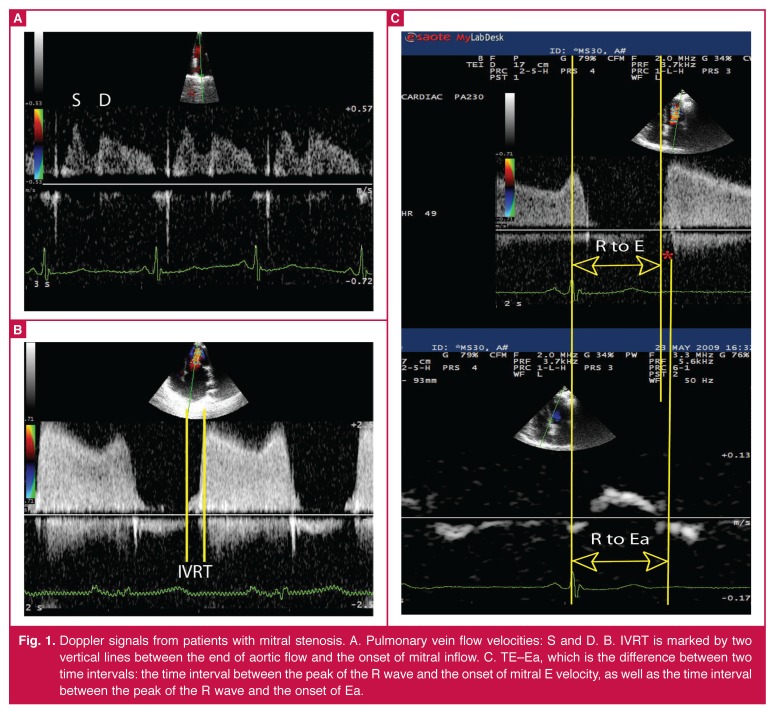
Doppler signals from patients with mitral stenosis. A. Pulmonary vein flow velocities: S and D. B. IVRT is marked by two vertical lines between the end of aortic flow and the onset of mitral inflow. C. TE–Ea, which is the difference between two time intervals: the time interval between the peak of the R wave and the onset of mitral E velocity, as well as the time interval between the peak of the R wave and the onset of Ea.
